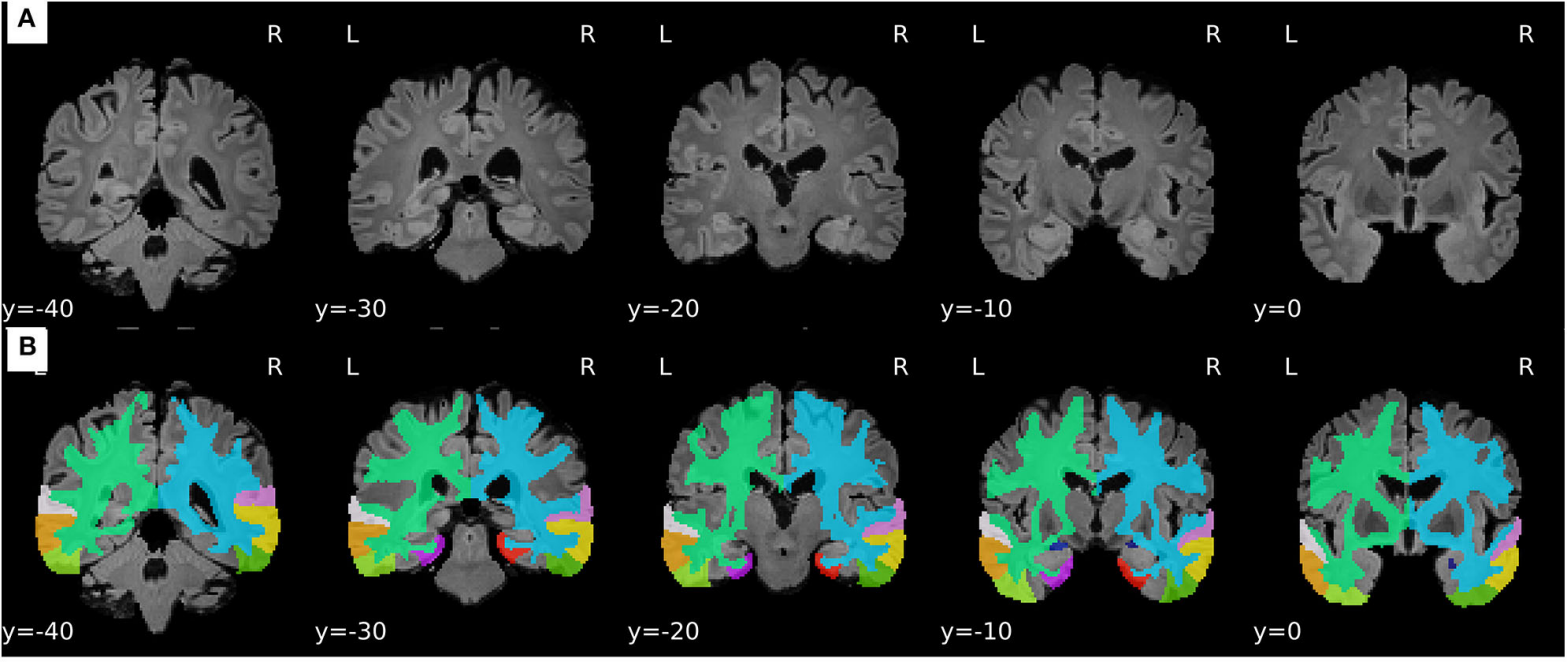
Representative samples of the pre-processing stage and feature extraction from FLAIR images. (A) Processed FLAIR images with a resolution of 1.5 × 1.5 × 1.5 mm. (B) Binary masks defined based on the selected ROIs.
